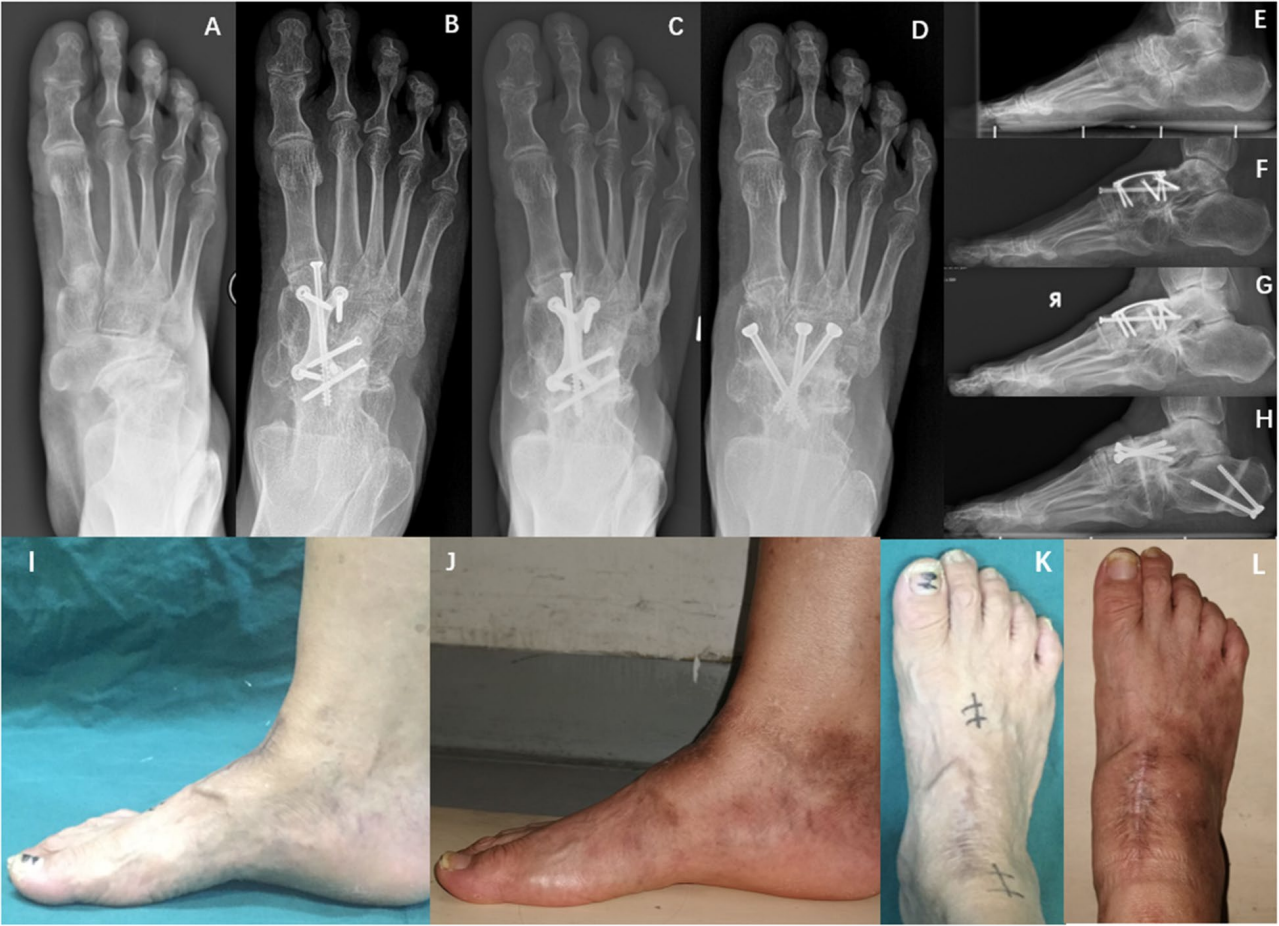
Patient No. 3. A-C Weight-bearing AP X-ray films before the operation, 3 months and 4 years after the operation. And mild forefoot supination deformity; D With midfoot osteotomy, final follow-up; E-H Weight-bearing lateral X-ray films before the operation, 3 months and 4 years after the operation, and last follow-up. And cooperating with calcaneal osteotomy to correct hindfoot varus; I-L Appearance of 4 years after operation and revision operation
